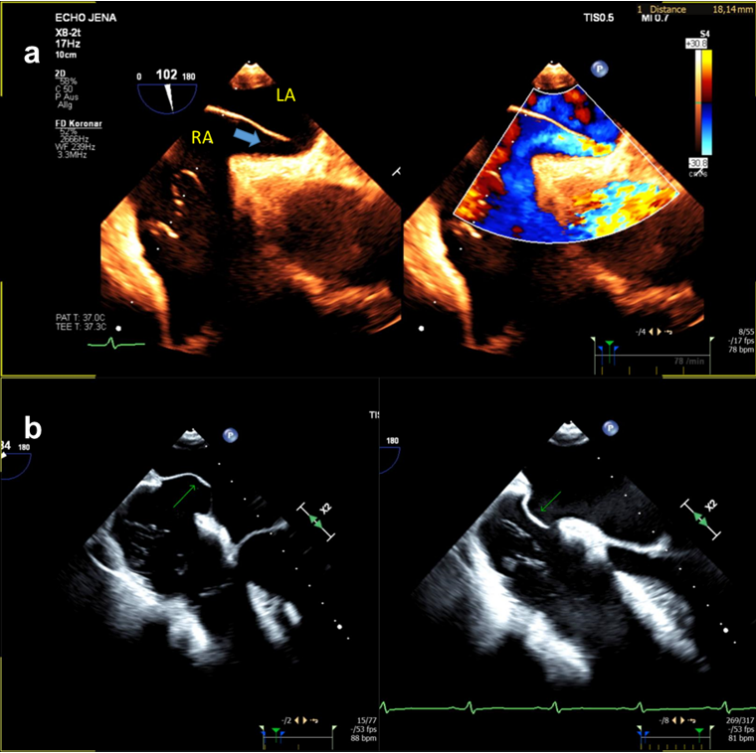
Different echocardiographic manifestations of high risk PFO. (a)
